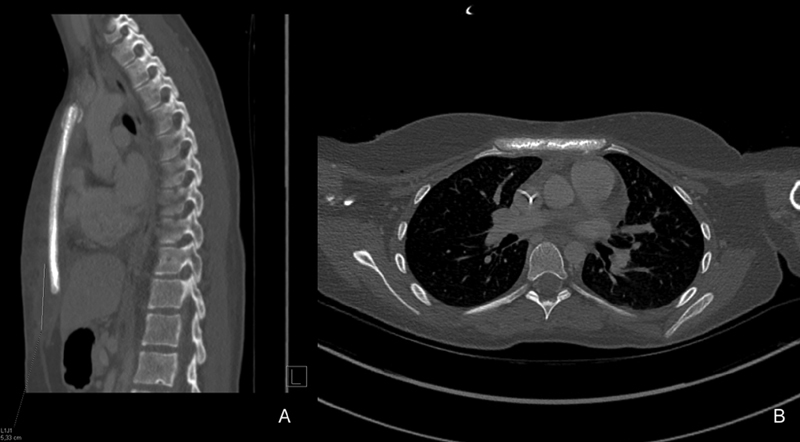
Three-week postoperative computed tomography scan.
